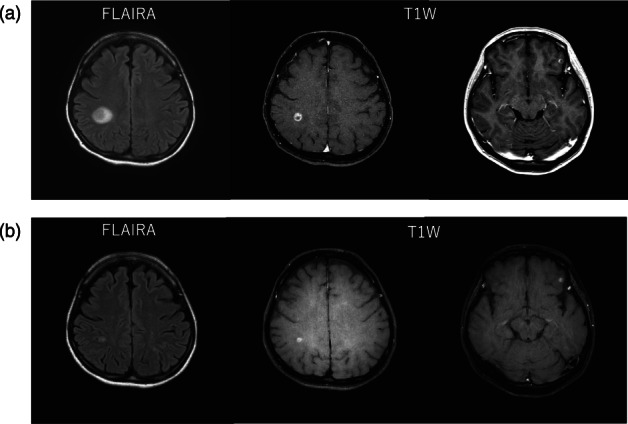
Head magnetic resonance imaging (MRI) (a) at initial diagnosis, and (b) 21 days after entrectinib administration.
